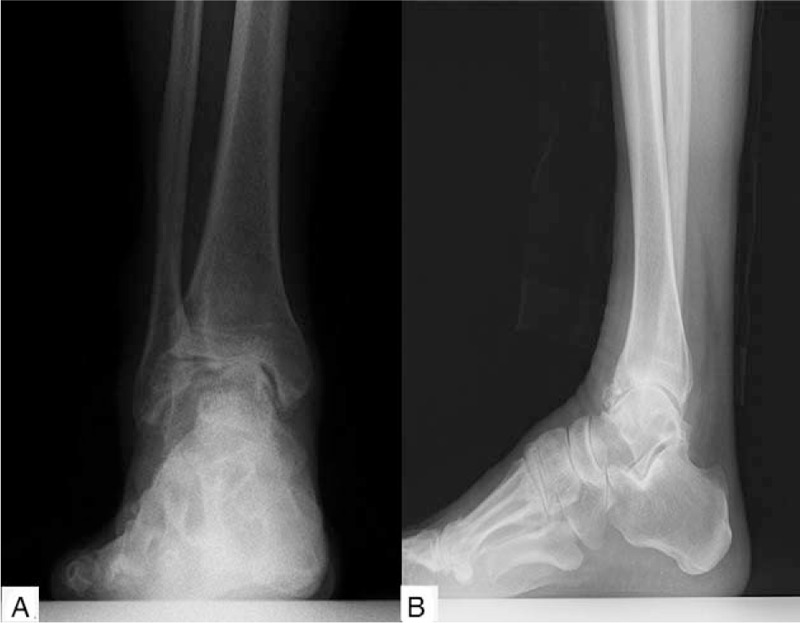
The weight-bearing positive (A) and lateral (B) radiograph of right ankle revealed many osteophytes and narrow joint space, especially in the medial side.
